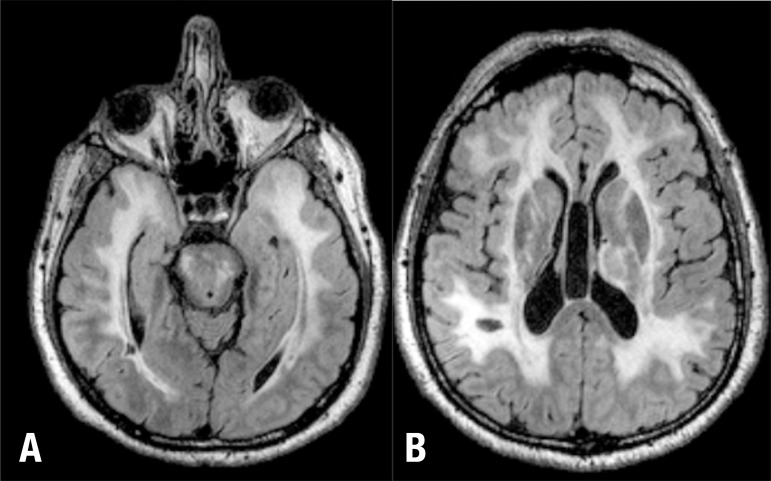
MRI FLAIR sequence in axial plan at temporal lobe [A] and basal ganglia
